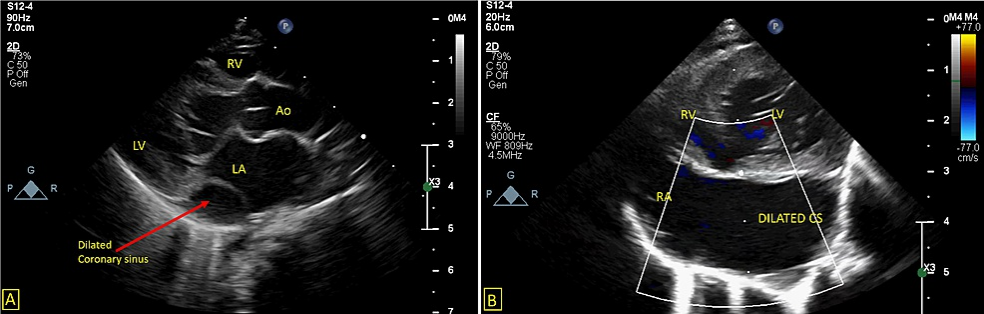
A: Parasternal long axis view showing a dilated coronary sinus. B: Foreshortened apical view showing a dilated coronary sinus. LA – Left atrium, LV – Left ventricle, RA – Right atrium, RV – Right ventricle, Ao – Aorta, CS – Coronary sinus
